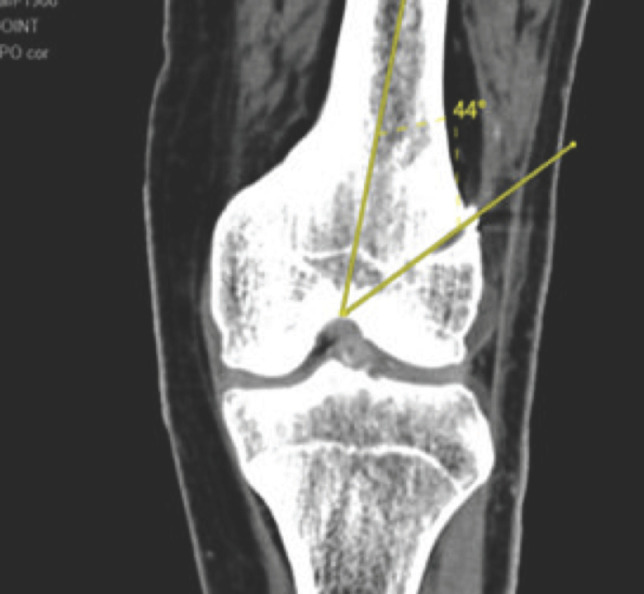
Coronal cut of the CT scan of knee joint showing measurement of the femoral tunnel angle.
